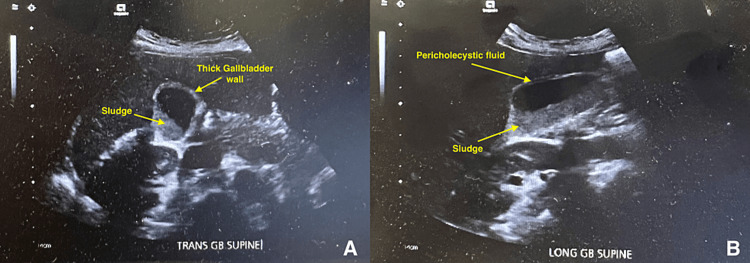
Abdominal ultrasound which shows mild gallbladder wall thickening and sludge
